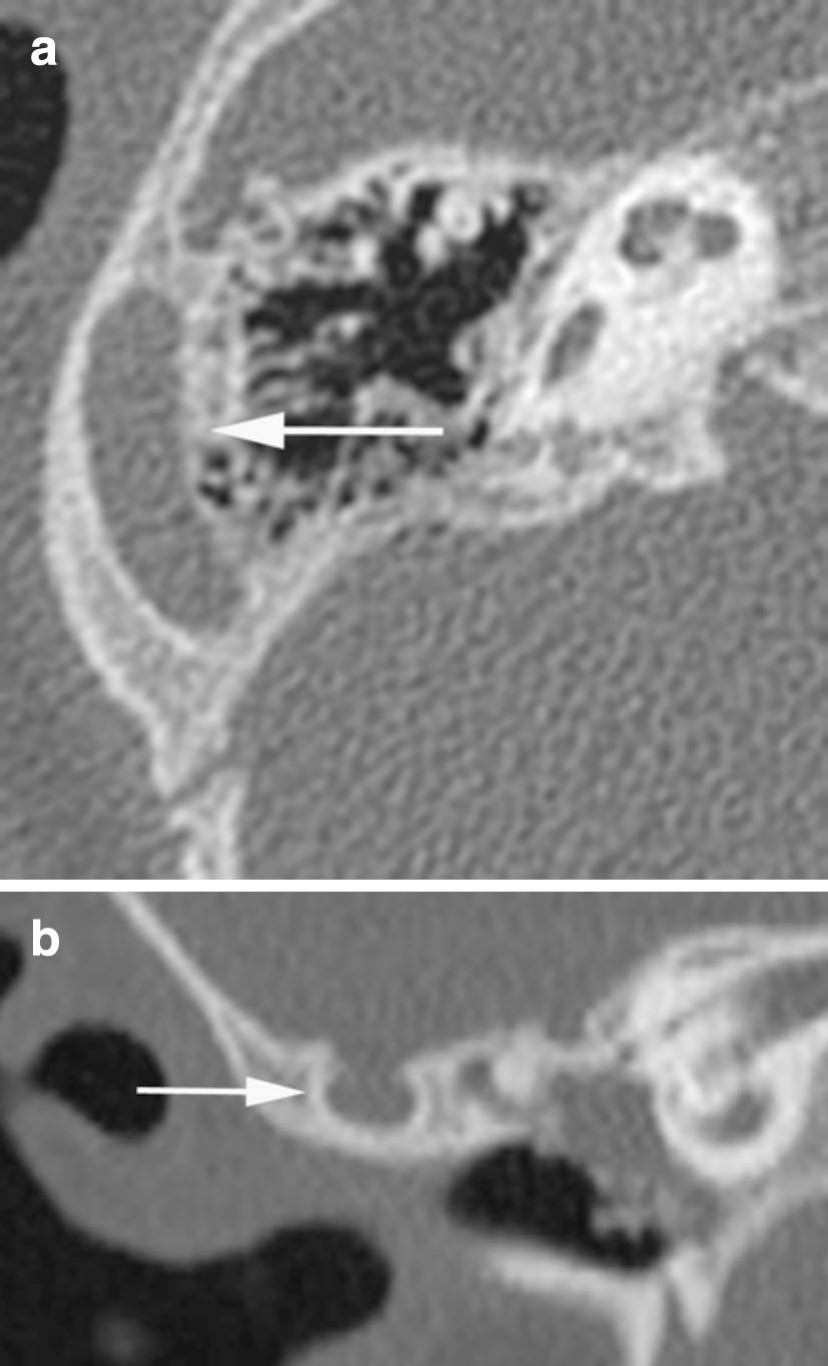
Petrosquamosal sinus. Axial (a) and coronal (b) CT image of a right ear showing this emissary vein coursing along the lateral superior surface of the temporal bone. The petrosquamosal sinus originates at the transverse sinus and drains either into the retromandibular vein or the pterygoid venous plexus
